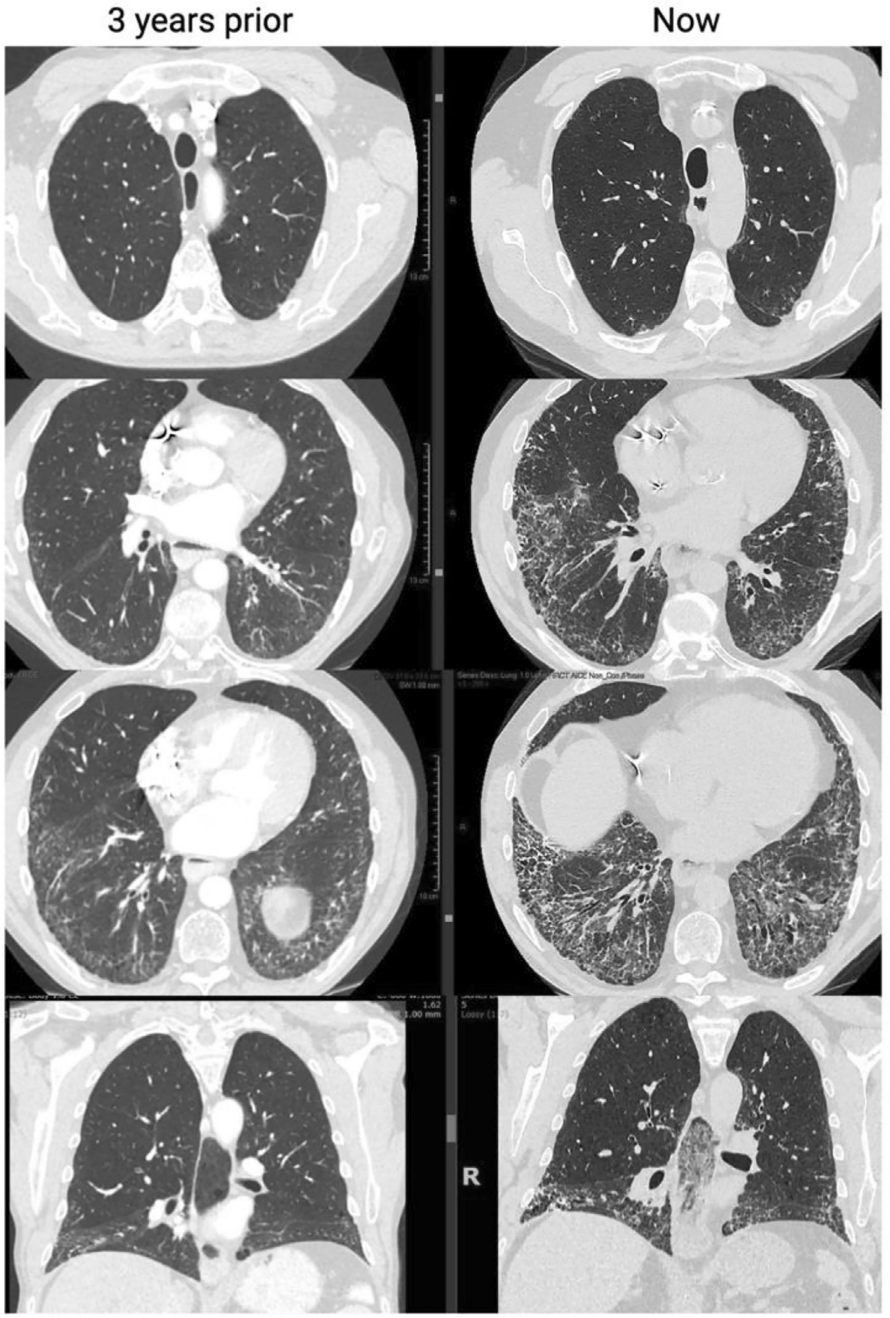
Chest high‐resolution computed tomography demonstrated progression from the scan three years prior. The scan remained in keeping in with NSIP, with ground glass opacification and reticulation alongside traction bronchiectasis and some freestanding bronchiectasis. The straight‐edge sign associated with NSIP was present. This radiologic feature is commonly reported with NSIP and less so with UIP interstitial lung disease.^27^ Diffuse dendriform pulmonary ossification, which is often associated with UIP and can also be found in NSIP, was seen.^28^ Subpleural sparing present on the previous scan was lost due to progressive fibrosis, with increased volume loss and progression of the morphology of fibrosis. Esophageal dilatation with fluid level is also demonstrated. NSIP, nonspecific interstitial pneumonia; UIP, usual interstitial pneumonia.
